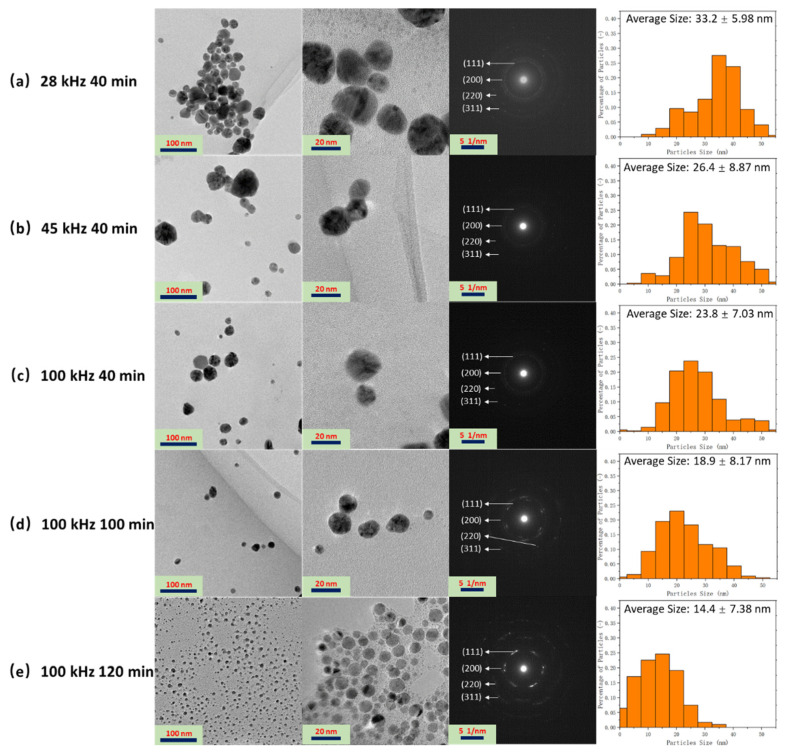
TEM images and SAED patterns of Ag NPs fabricated at different ultrasonic irradiation frequencies (28, 45, and 100 kHz) and durations (40, 80, and 120 min), (a) 28 kHz 40 min; (b) 45 kHz 40 min; (c) 100 kHz 40 min; (d) 100 kHz 100 min; (e) 100 kHz 120 min.
